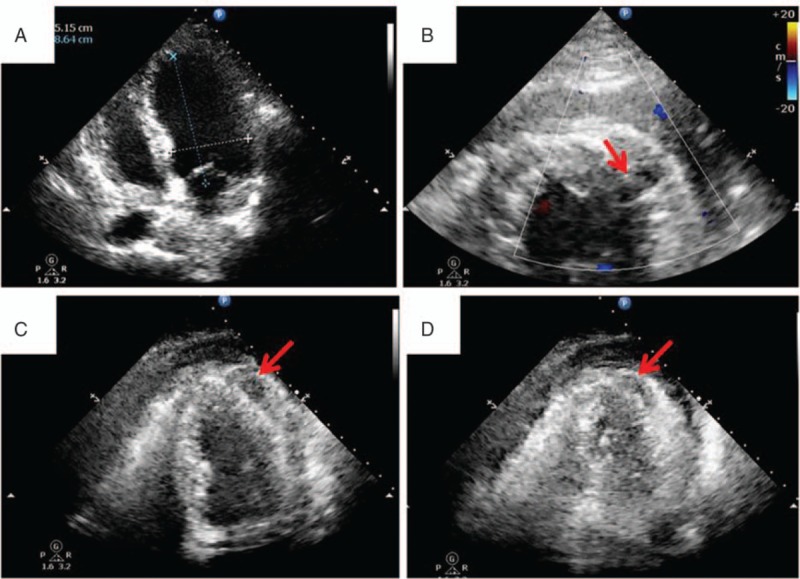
Preoperative cardiac images, (A) transthoracic echocardiography before the radiofrequency catheter ablation (RFCA), (B) transthoracic echocardiography after the RFCA (red arrow: rupture of the left ventricular wall), (C) transthoracic echocardiography after the RFCA (red arrow: hematoma in the left ventricular wall during diastole), (D) transthoracic echocardiography after the RFCA (red arrow: hematoma in the left ventricular wall during systole: smaller than that during diastole due to the contraction of the myocardium).
